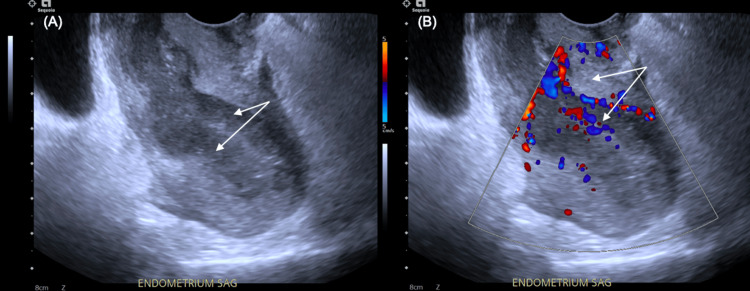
Transvaginal ultrasound, sagittal view of the uterus. (A) Transvaginal ultrasound demonstrates an 8-cm retroflexed uterus with increased thickness of the sub-endometrial hypoechoic halo (arrows). (B) Color Doppler demonstrates areas of increased vascularity and small cysts within the endometrium (arrows).
